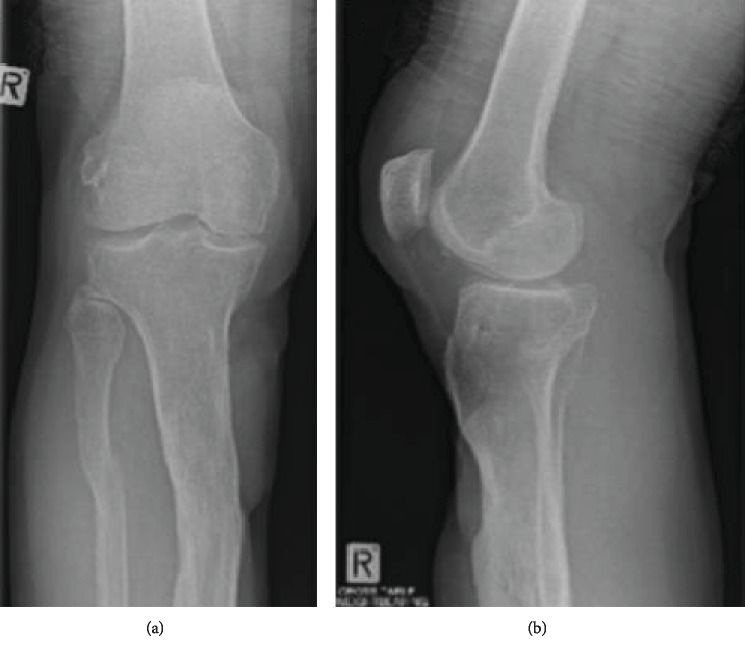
Anteroposterior and lateral radiographs showing the healed proximal tibia fracture and posterior subluxation of the tibia. Weight-bearing AP (a) and lateral (b) radiographs of MR AD showing evidence of a healed proximal tibia and fibula fracture and posterior subluxation of the tibia on the femur. Importantly, the posterior slope of his proximal tibia is within normal limits measuring 6 degrees.
